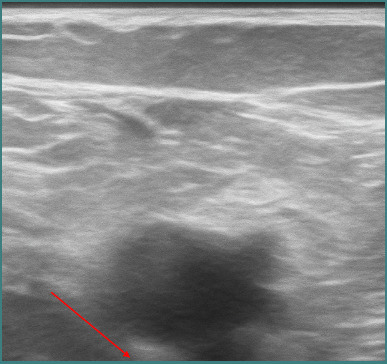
Venous Doppler ultrasound
